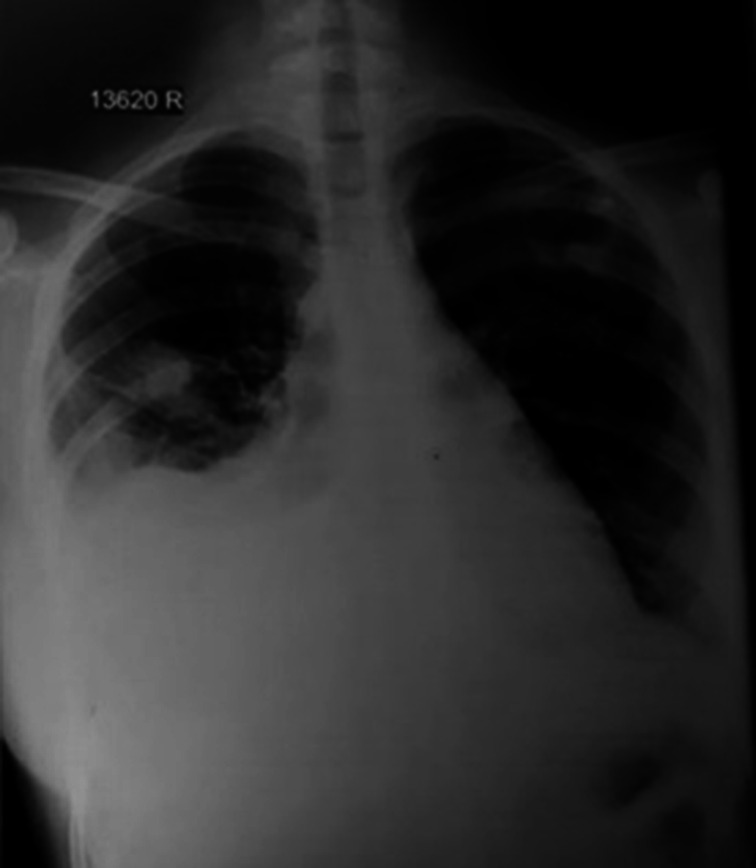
Chest radiograph-bilateral pleural effusion with opacification in the right mid-zone.
